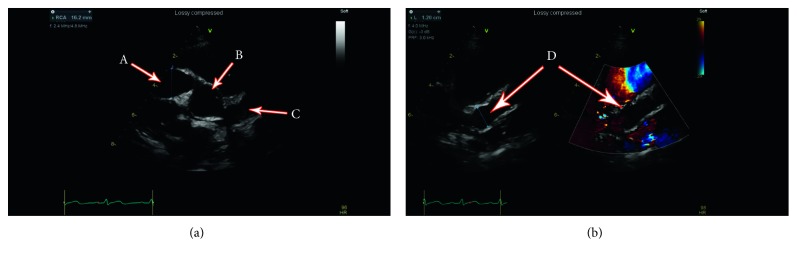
Echocardiogram: (a) Massive ectasia and aneurysmal dilatation of the right coronary artery (16 mm × 17 mm) (A), aorta (B), and left coronary artery (C). (b) Massive ectasia and aneurysmal dilatation of left main artery (13 mm × 13 mm) (D).
